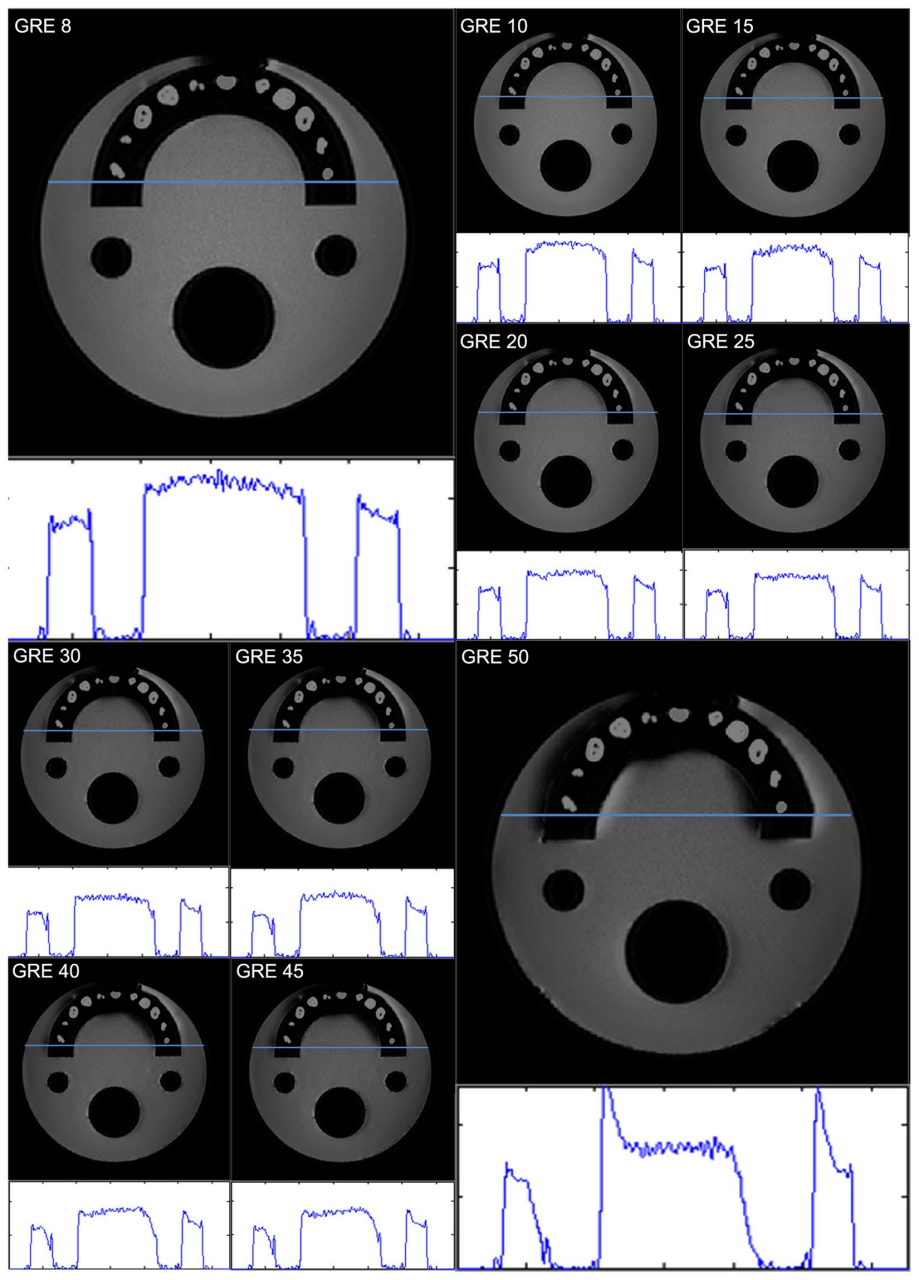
The signal intensity distribution on the line that drawn on the CT/MR registered image. The teeth are segmented from the top middle dental restoration CT image and registered on MR images with difference pulse sequences (3.0 T). Figures acquired comparison of signal intensity with different GRE sequences with same segmentation region registered.
